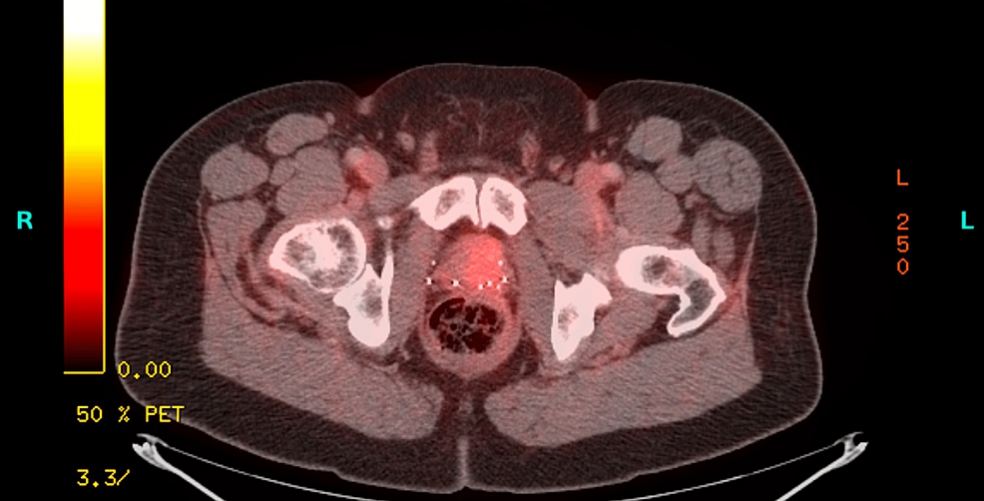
PET-CT, a selected axial cross-section at the level of the prostate from July 8, 2022
